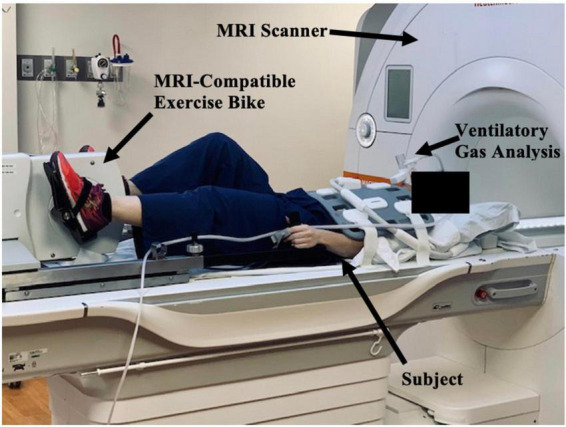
Study set-up with magnetic resonance imaging (MRI)-compatible exercise bike positioned in CMR imaging scanner. The subject, MRI scanner, MRI-compatible exercise bike, and ventilatory gas analysis are indicated by black arrows. The subject lies outside the MRI scanner and will pedal on an exercise bike as they move through the scanner. Ventilatory gas analysis is simultaneously performed while a cardiac MRI is obtained.
